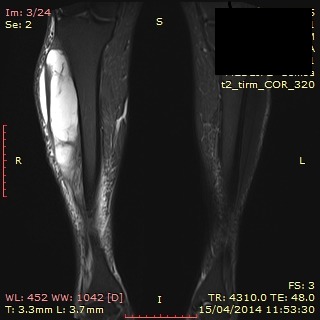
Gadolinum enhanced MRI showing significant tumor reduction (Personal photographic archive)
